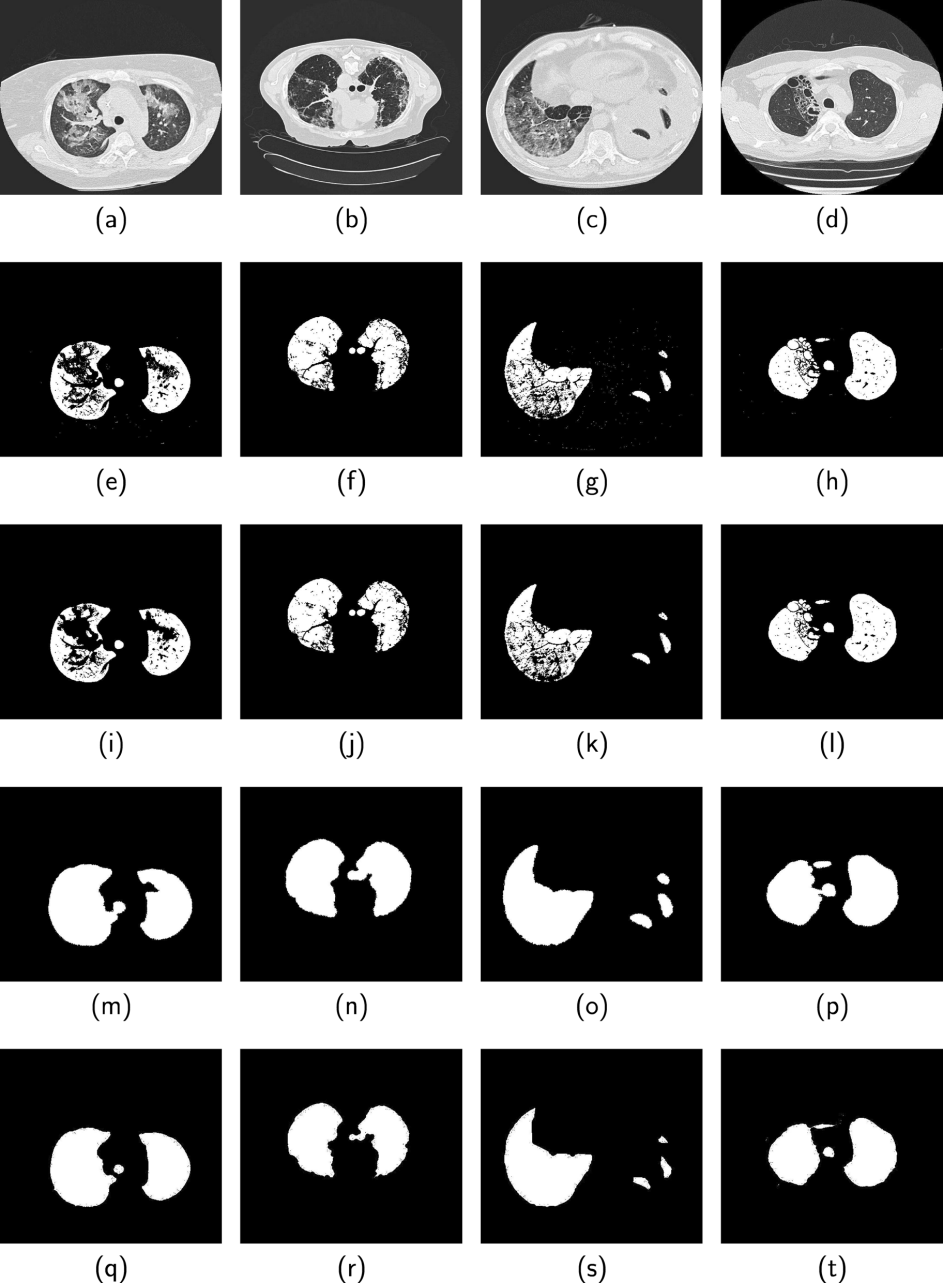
Performance of the proposed method on four CT images from the ILD database are displayed in columns 1, 2, 3 and 4. (Row 1) Original image, (Row 2) Binarized image, (Row 3) Candidate lung region, (Row 4) Segmented lung region (Row 5) Groundtruth
